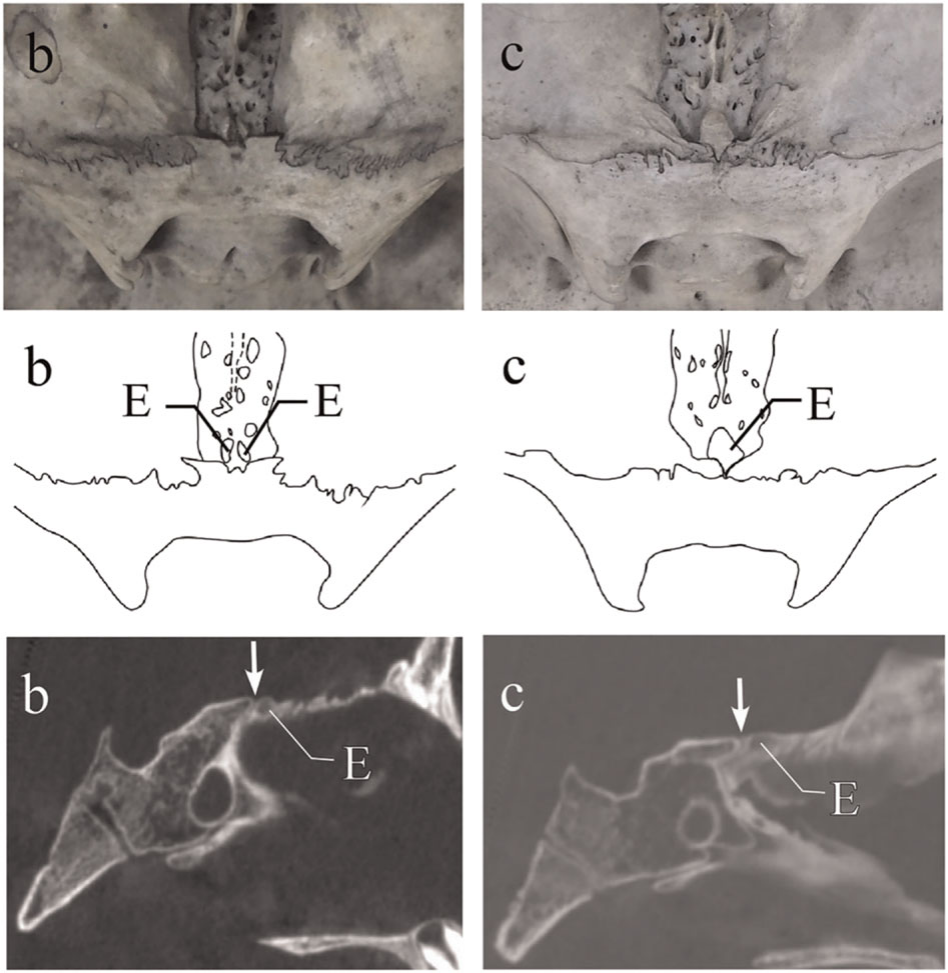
Morphological variations of the anterior cranial fossa in late childhood (7 years). Upper row shows endocranial photographs, middle row shows traced images of photographs, and lower row shows micro‐CT images in the median sagittal plane. (b) Median part of the sphenoid body projects slightly anteriorly, and anterior border of the protrusion has a straight outline. (c) Median part of the sphenoid body does not project anteriorly. Orbital plates of the right and left sides of frontal bones are close to each other in the median part. E, ethmoid bone. Arrows show spheno‐ethmoidal suture on CT images. CT: computed tomography
